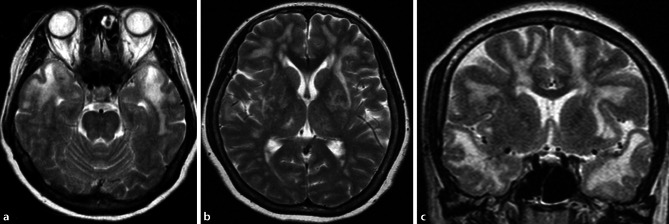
CADASIL. A 50-year-old female patient with CADASIL who presented with characteristic extensive white matter hyperintensities, particularly bilateral temporal, depicted on axial (a, b) and coronal (c) T2-weighted images
